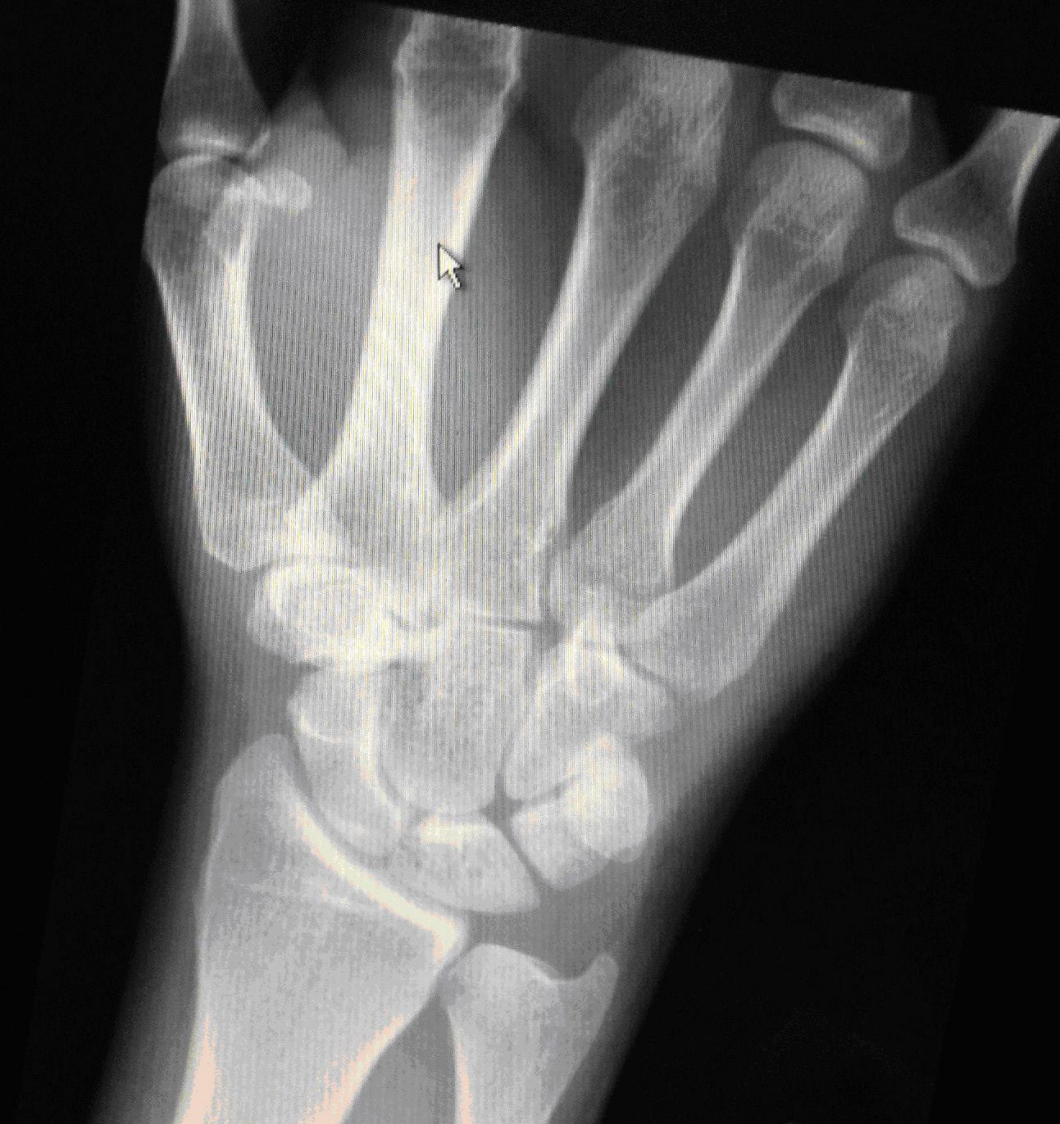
Posteroanterior plain radiograph of the right wrist at six weeks after the injury. The image shows no obvious evidence of bony injury.
